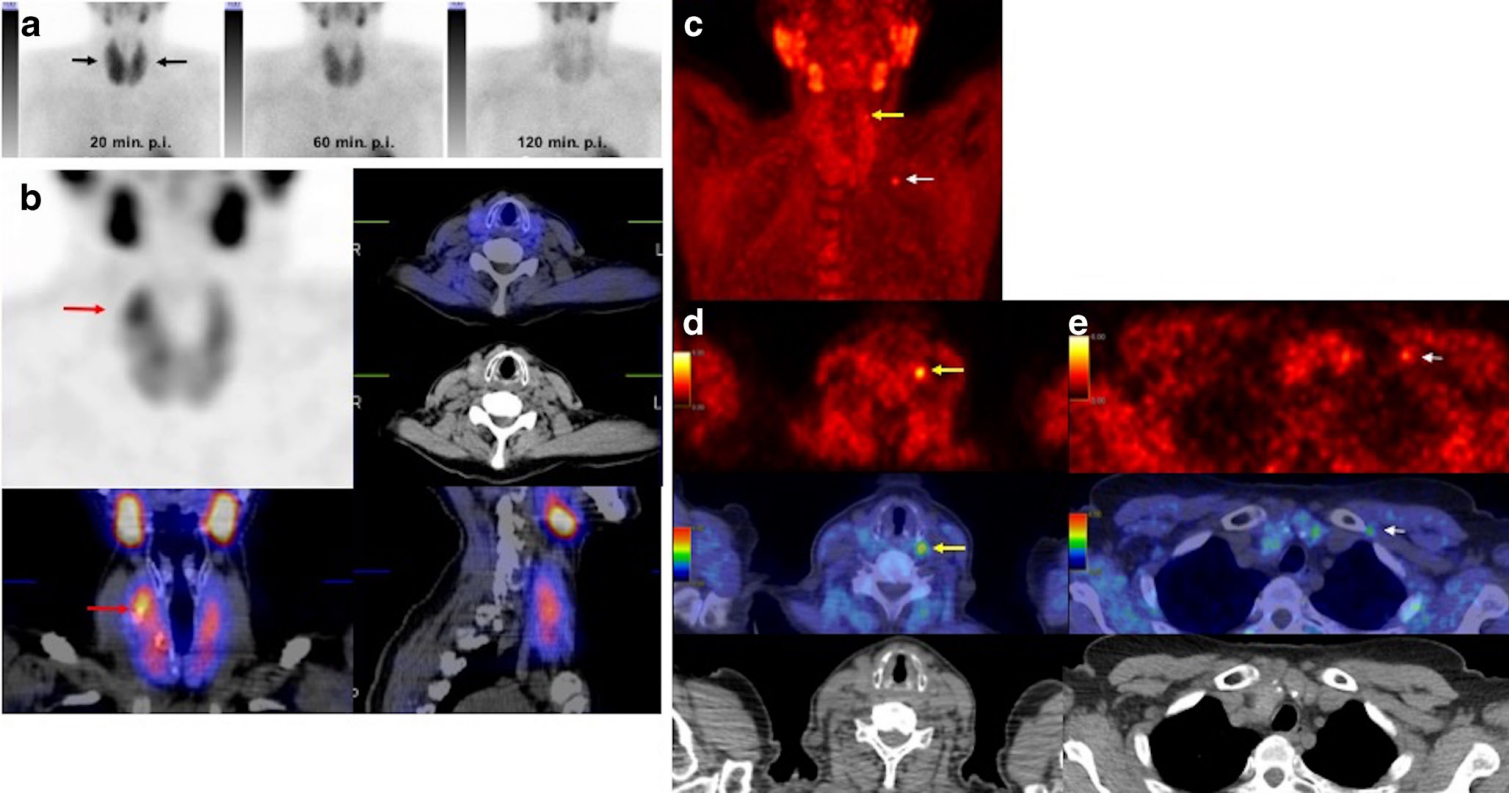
^99m^Tc-MIBI planar scintigraphy images (a), ^99m^Tc-MIBI SPECT/CT images (b) and ^18^F-fluorocholine PET/CT images (c–e) in a 48-year-old patient with elevated serum PTH and calcium levels of 91.29 pg/ml and 2.87 mmol/l, respectively. a Anterior ^99m^Tc-MIBI planar images show diffuse nonhomogeneous tracer uptake in the enlarged thyroid gland in the image 20 min after injection (20 min p.i. *arrows*) with regular wash-in seen on the 60-min image (60 min p.i.) and 120-min image (120 min p.i.) without any evidence of focal tracer retention suggestive of parathyroid adenoma. b
